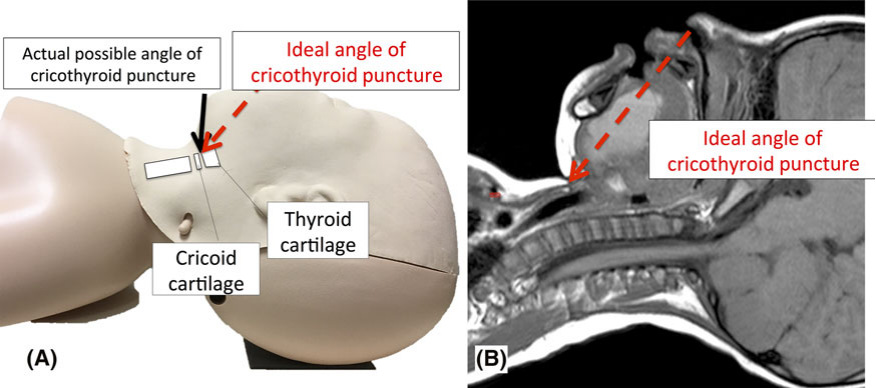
Treatment of pediatric “cannot intubate, cannot oxygenate” cases. A, Model of the approach used in percutaneous cannula cricothyroidotomy. Neck extension in small children does not provide a sufficient angle to safely perform percutaneous cricothyroidotomy. B, Magnetic resonance image of a 3‐year‐old boy whose case of “cannot intubate, cannot oxygenate” is reported of here. This image shows insufficient working space or angle to safely perform percutaneous cricothyroidotomy.
